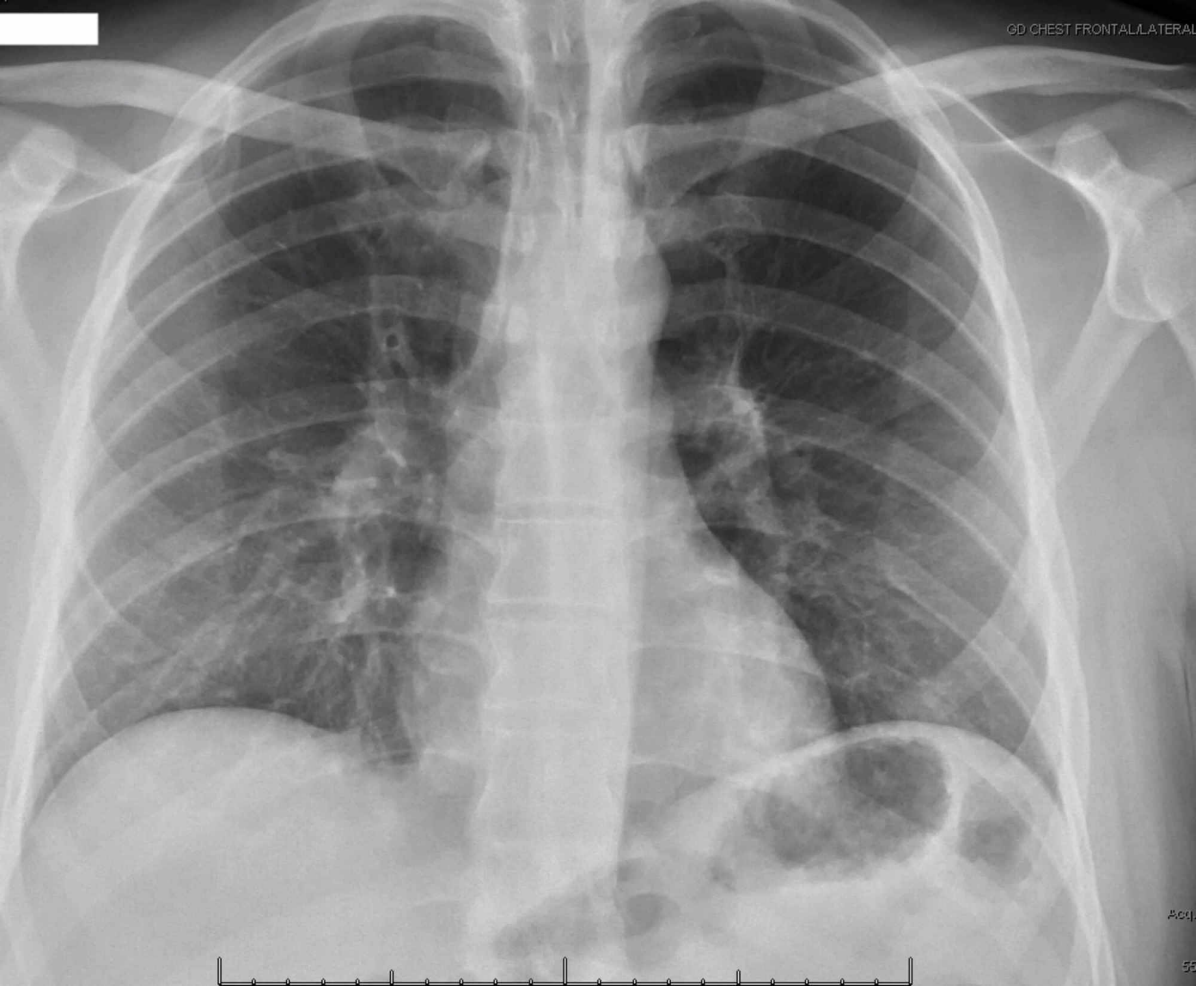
Normal chest X-ray. A chest X-ray revealed no significant abnormalities during fever work-up.
